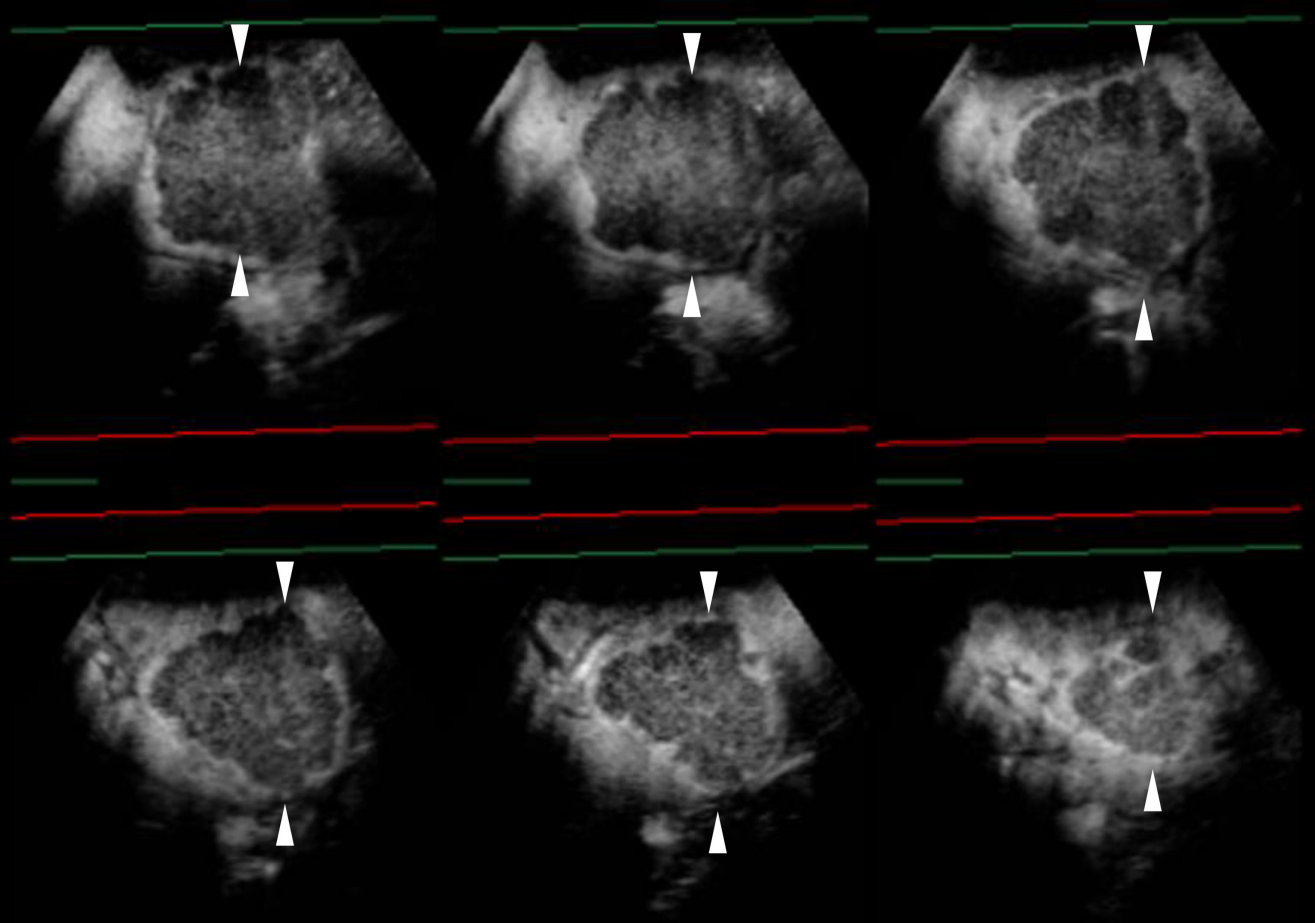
Sonazoid-enhanced ultrasound (US) images of a man in his seventies with a pathological diagnosis of moderately differentiated hepatocellular carcinoma (HCC), who had a history of hepatitis C. The tumor was 70mm. Consecutive lateral images of the tumor remarkably illumed the irregular margin on the three-dimensional (3D) US, which was obtained by auto-sweep 3D scanning in the post-vascular phase. Tomographic ultrasound images in plane A, which can be translated from front to rear, with a slice distance of 4.8 mm. Arrowheads indicate the margin of the HCC lesion.
